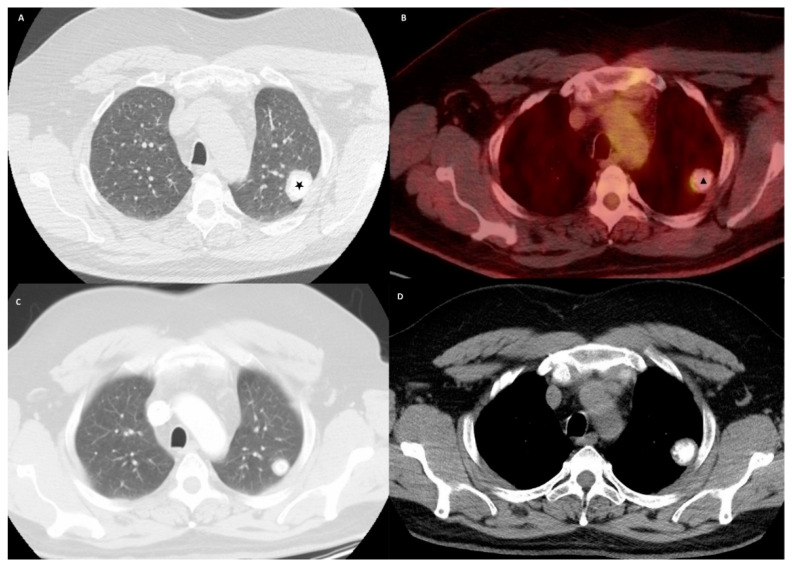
(A) is a computed tomography of chest in an axial plane showing a pleural based left upper lobe mass (denoted by a star), (B) is a positron emission tomography of chest in an axial plane showing mild avidity in the left upper lobe mass, (C) is computed tomography of chest in axial plane showing left upper lobe nodule, which is smaller in size ten years back, and (D) shows the left upper lobe nodule with central calcification.
